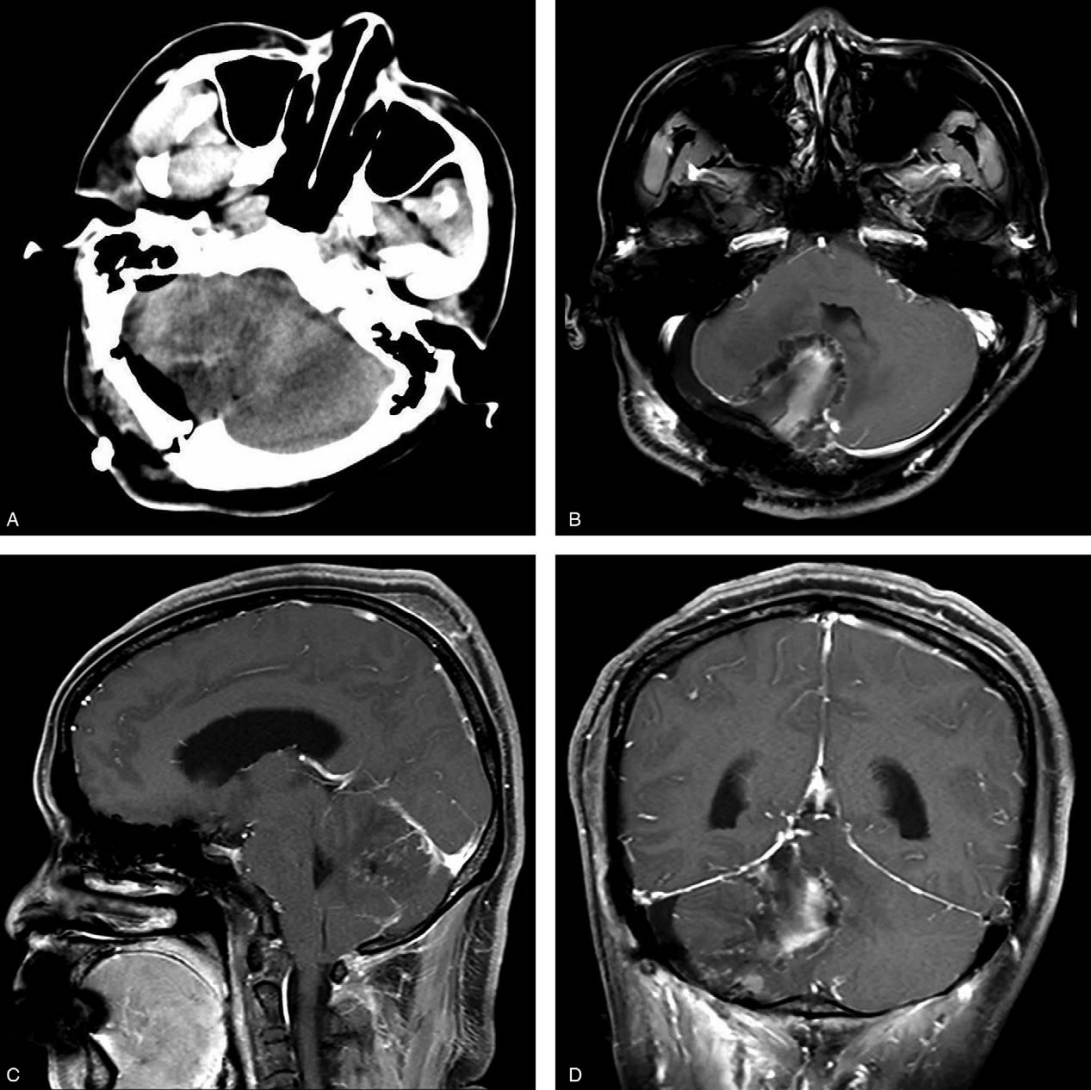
(A–D): Postoperative CT scan and MRIs showing total resection of the tumor. A, CT scan; B, axial; C, sagittal; D, coronary. CT = computed tomography, MRI = magnetic resonance imaging.
